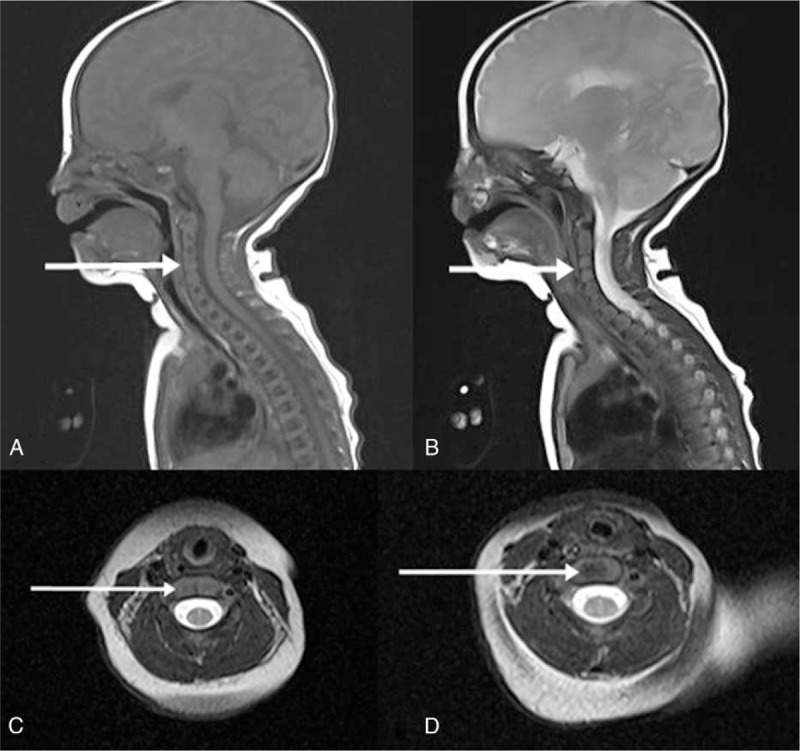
Female patient with Wolf-Hirschhorn syndrome. Cervical spine magnetic resonance imaging at 1 month of age. (A) T1-weighted sagittal view, (B) T2-weighted sagittal view. (C) T2-weighted axial view at C4 level, (D) T2-weighted axial view at C6 level. The hypointense lesions (arrows) in the vertebral body indicate the invisible ossification centers in C3 and C4.
